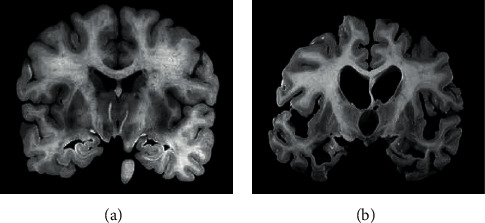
MRI images of normal people and AD patients.
